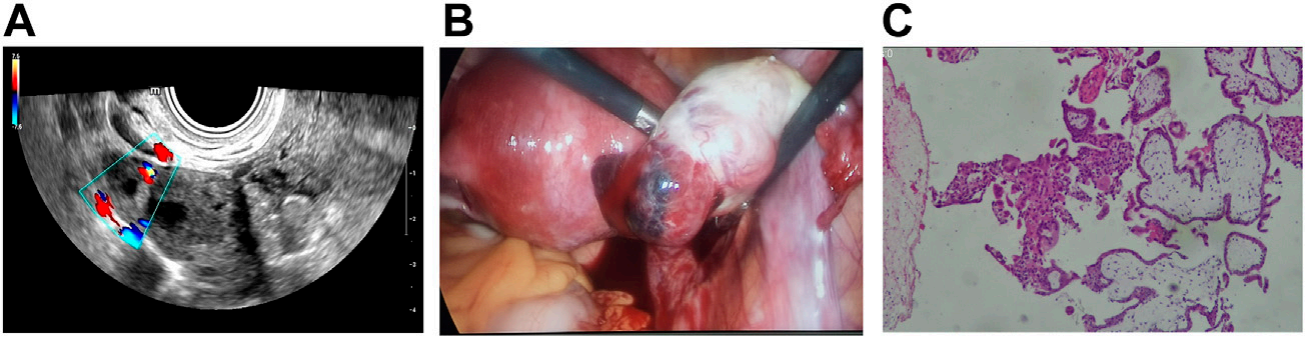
(A) Transvaginal ultrasonography revealed an echogenic mass in the right ovary. (B) Ovarian pregnancy was detected using a laparoscope. (C) Postoperative pathological analysis confirmed right ovarian gestation, revealing both chorionic villi and trophoblasts.
